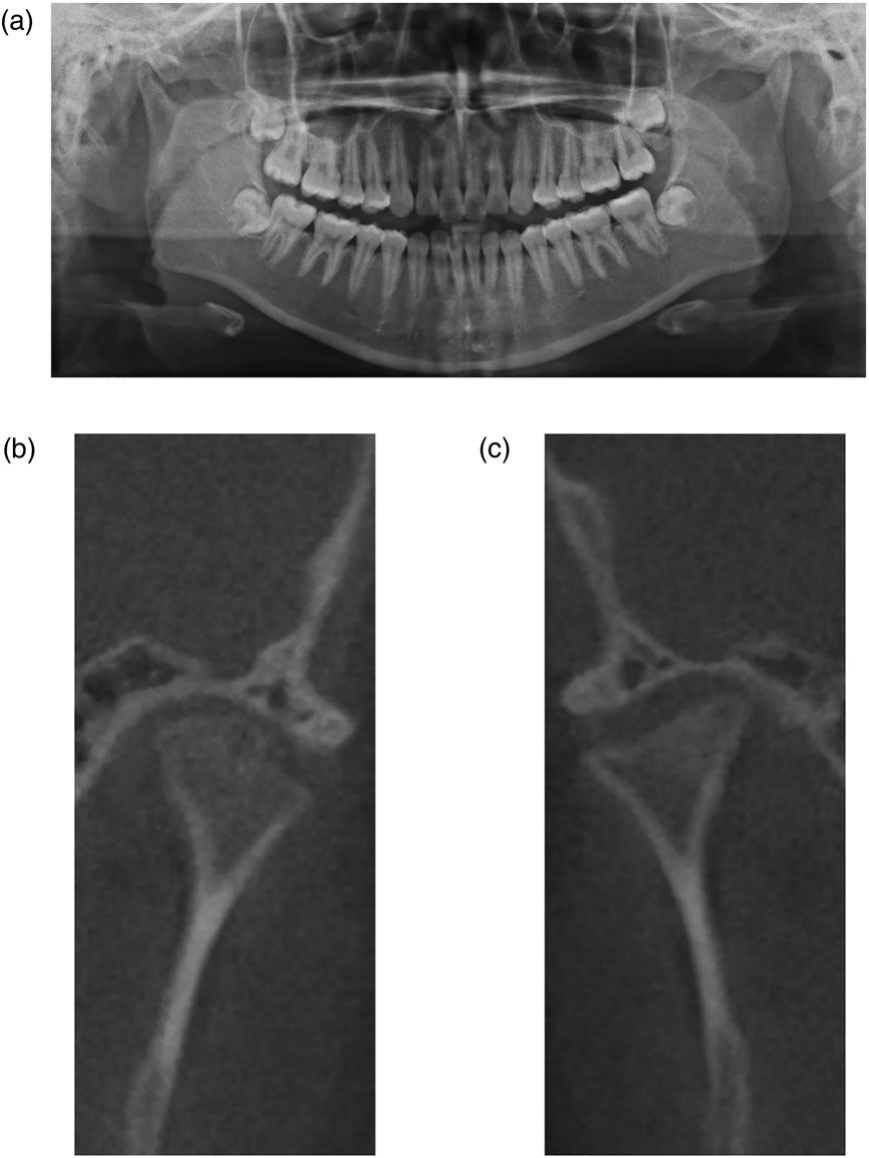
Visual diagnosis of temporomandibular joint osteoarthritis with panoramic radiography (PR) and cone beam computed tomography (CBCT) images. (a) A representative PR image of a 14‐year‐old female with temporomandibular disorder symptoms. A coronal CBCT for the same patient showed erosive bone change, sclerosis, flattening on the right condyle (b), and surface irregularity of the left condyle (c)
